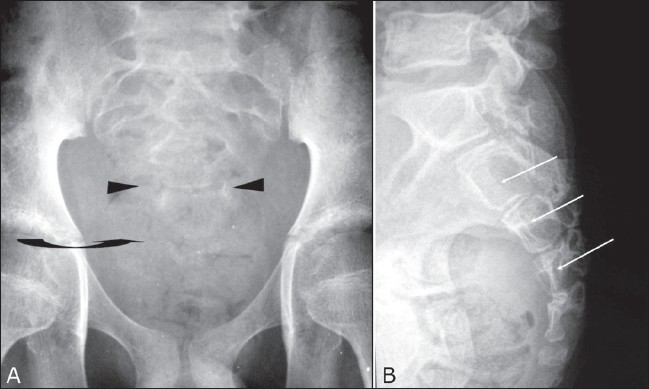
Anteroposterior (A) and lateral (B) plain radiographs of the lumbosacral (LS) spine reveal posterior scalloping of the sacral vertebrae (S2–S4) (arrows), widened and ill-defined sacral foramina (arrowheads), and an overlying right-sided soft tissue haze (curved arrow)
